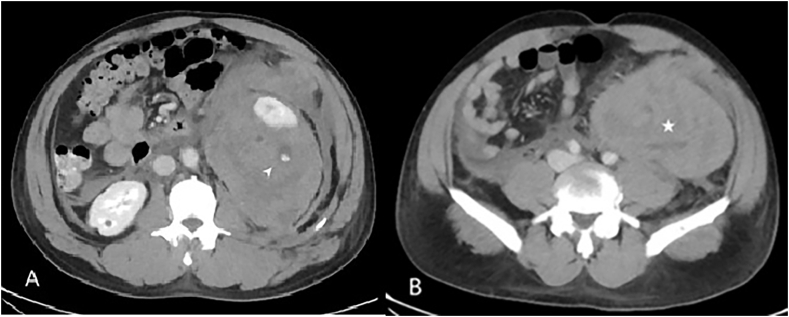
(a) The subsequent CT demonstrates an increase in the size of the hematoma, and arterial contrast extravasation was detected (arrow). (b) The hematoma had expanded and extended into the pelvis.
